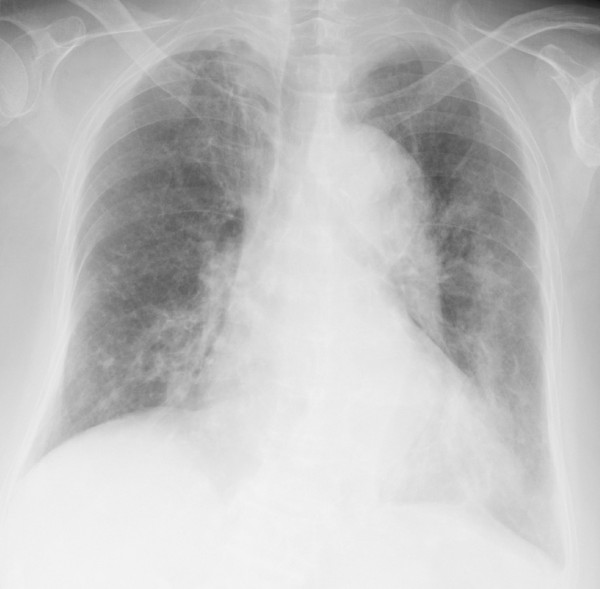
Chest X-ray obtained on admission showed consolidation in the left middle and lower lung fields.
